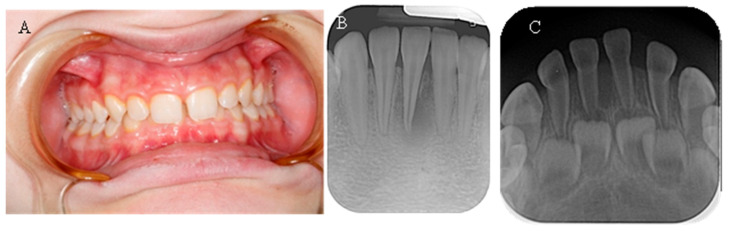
(A) Intraoral image of a 17-year-old girl with XLH; (B) Intraoral X-ray of the same girl with visible necrosis of an anterior teeth without previous trauma history; (C) Intraoral X-ray of a 2.5-year-old girl with XLH. Thinner radicular dentin is clearly visible.
